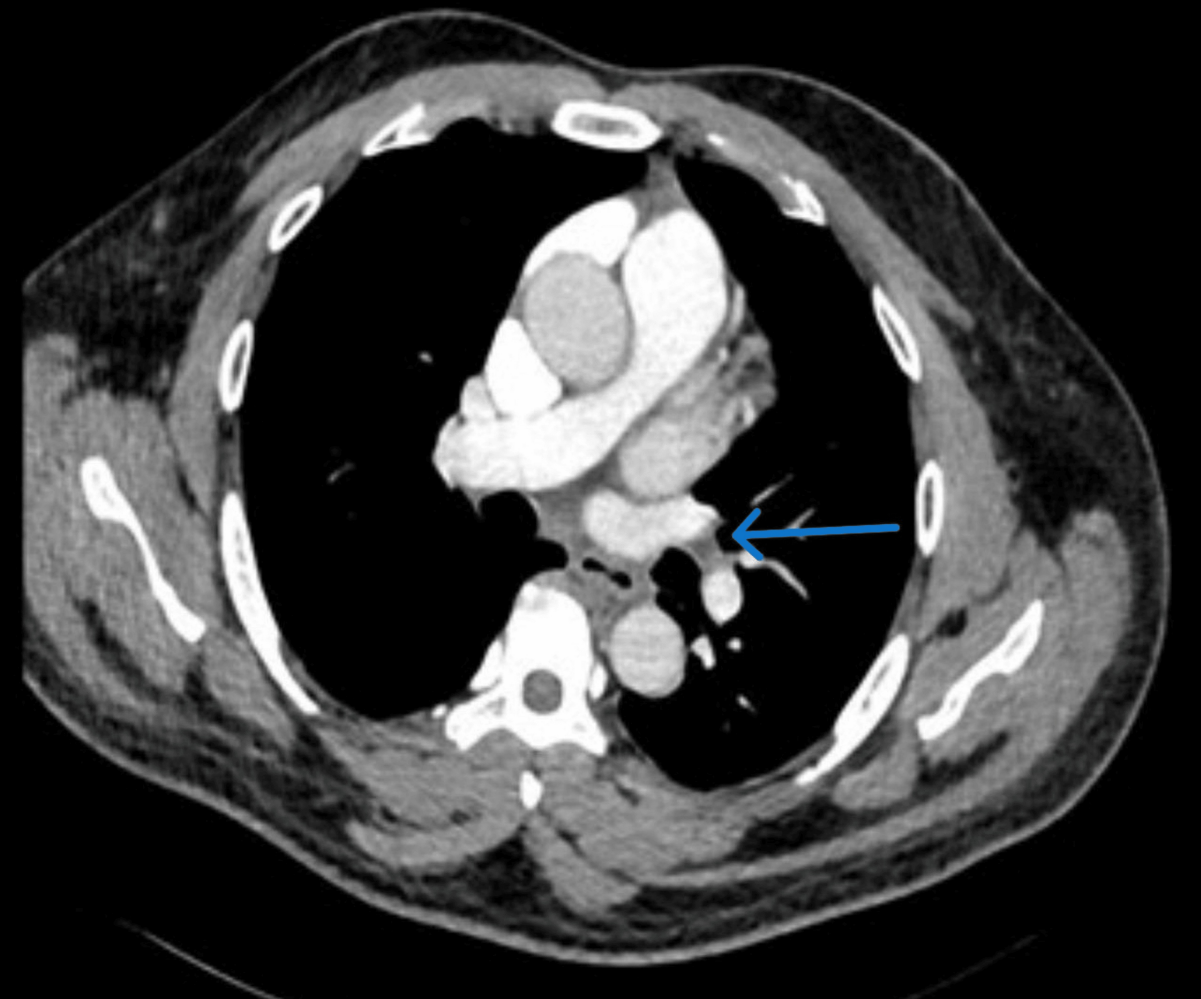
Computed tomography pulmonary angiogram showing bilateral partial filling defect (blue arrow) indicating pulmonary embolism (left side)
